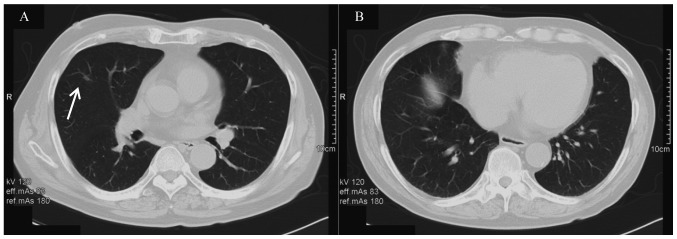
Chest computed tomography following six courses of therapy. (A) The tumor in the right lung has become scar tissue (arrow); and (B) no metastasis is observable in the left lung.
